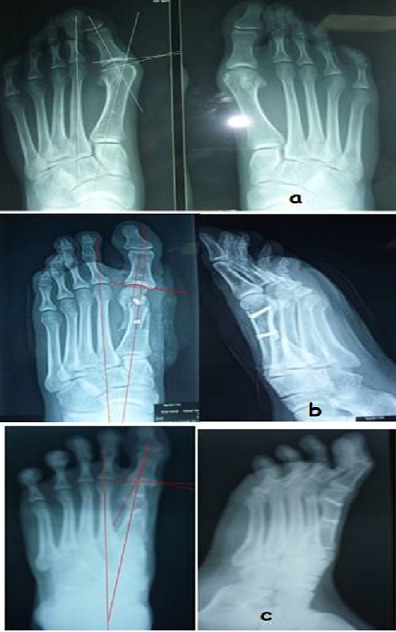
Résultats anatomiques de l'observation n°11 (B.0); a) aspect radiologique préopératoire; b) radiographie standard postopératoire immédiate; c) contrôle radiologique au recul d'un an
